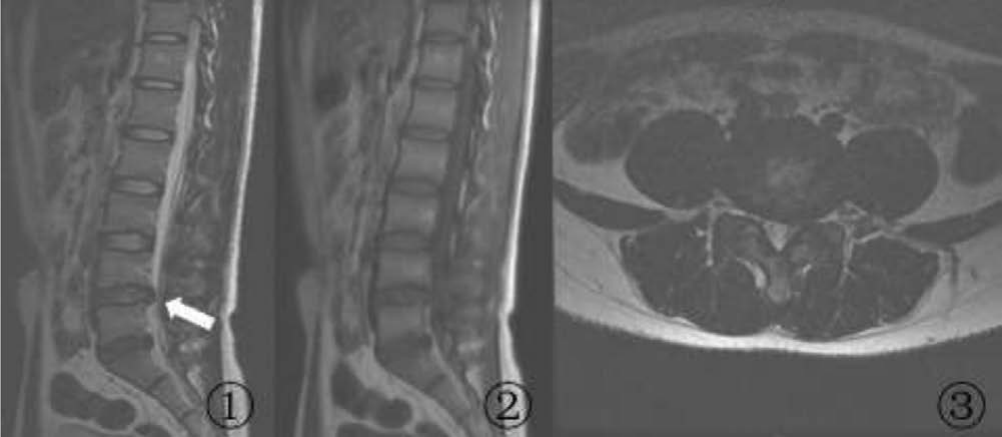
Preoperative lumbar MRI. A (A) sagittal T2-weighted image, (B) sagittal T1-weighted image, and (C) transverse T2-weighted image show the L4/5 disc protruding posteriorly and to the left, and compressing the left L5 nerve root. MRI = magnetic resonance imaging.
